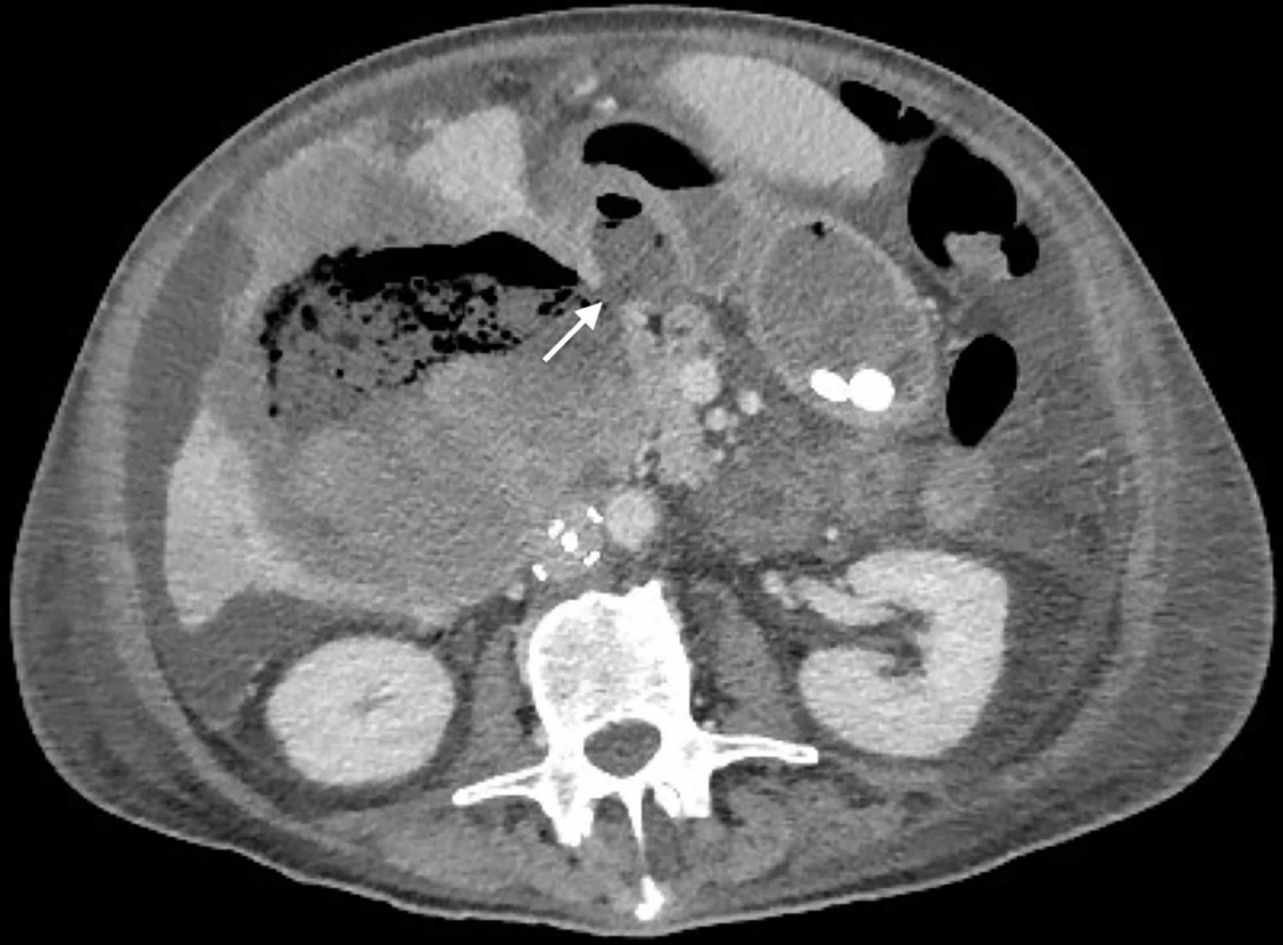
Coronal view of abdominal CT showing a large mass involving the central liver. The arrow shows the mass fistulizing to the duodenum.
